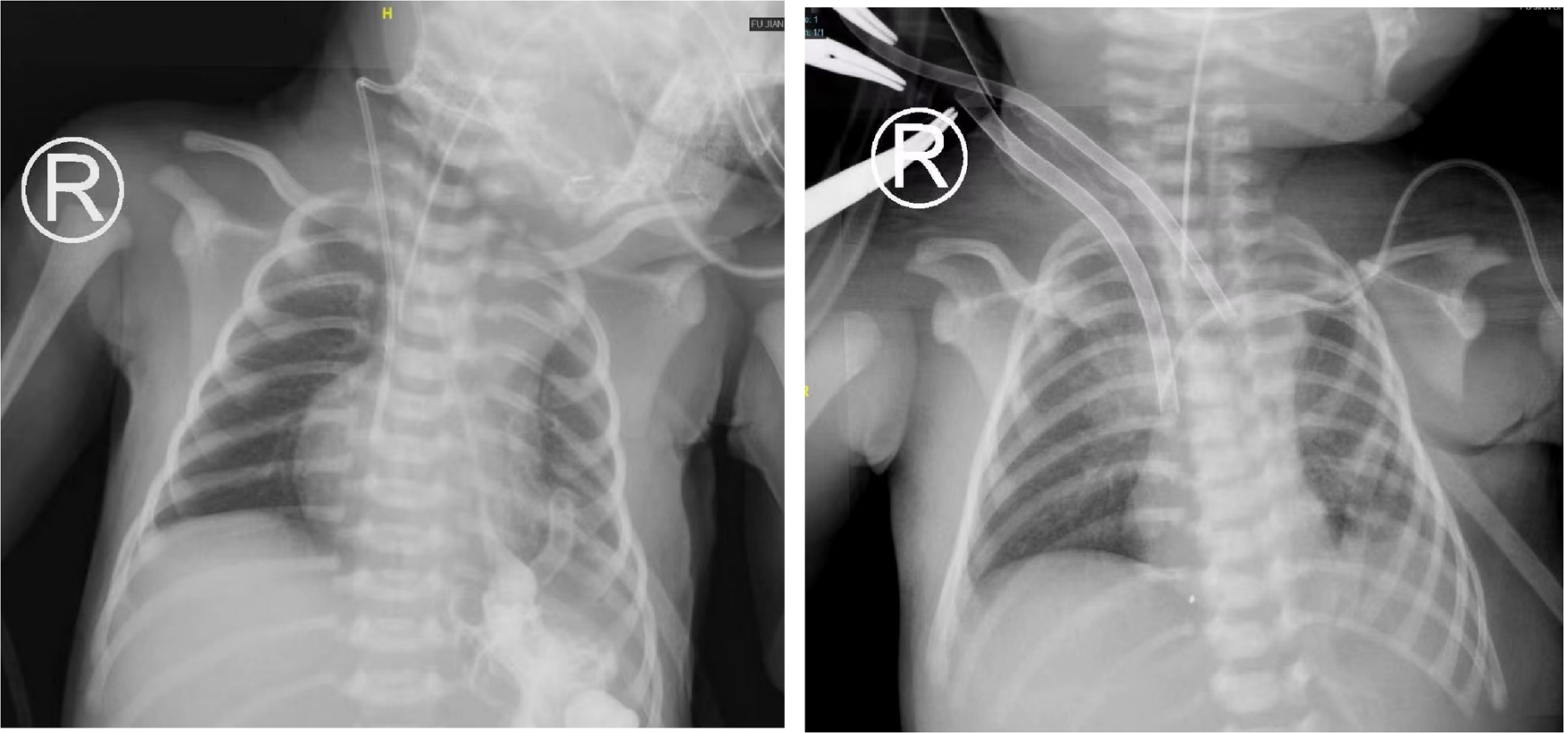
x-ray before ECMO treatment and during ECMO treatment in case 1.
